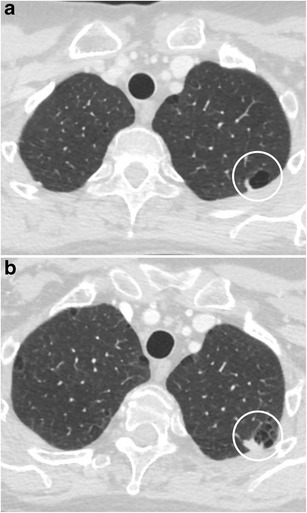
A 51-year-old woman with myelitis transversa presented on CT with a cystic airspace with mural nodule. Axial CT-image in lung window setting a shows a subpleural cystic airspace with a mural nodule with somewhat thin, bandlike morphology. Follow-up CT 6 months later b shows increase in size of the nodular component and more multicystic aspect of the cystic airspace. The lesion was found suspicious for a lung cancer associated with cystic airspaces. Lobectomy was performed and confirmed the malignant aetiology showing a poorly differentiated adenocarcinoma
